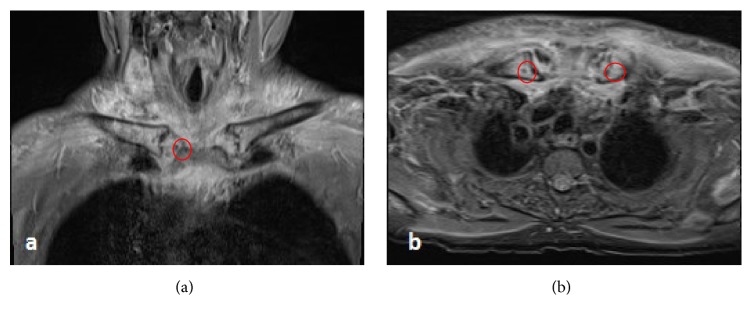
Sternoclavicular joints MRI scan STIR sequence. (a) Coronal image; (b) axial image showing moderate bone marrow edema in the medial third of the clavicles bilaterally, extending to the subarticular region. There was fluid signal in the SCJ space with mild to moderate marrow edema seen in the manubrium. No radiological signs of osteomyelitis were noted.
